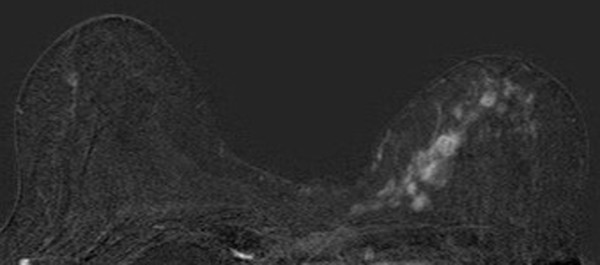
Invasive lobular carcinoma in a 67-year-old woman with left nipple stiffness and retraction. The contrast-enhanced transaxial breast magnetic resonance image shows the same non-mass regional enhancement than CEDM images.
